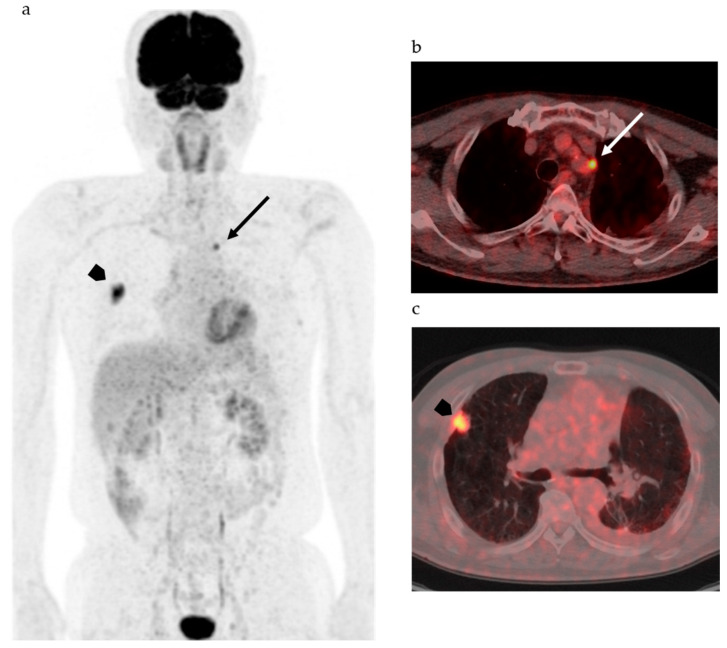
2-[^18^F] fluoro-2-deoxy-d-glucose positron emission tomography/computed tomography (FDG-PET/CT) of patients with non-small cell lung cancer. (a) Maximum intense projection image shows abnormal FDG uptake in the left upper mediastinum (arrow) and the right lung (arrowhead). (b) PET/CT shows increased FDG uptake in the lymph node adjacent to the left subclavian artery at the level of the upper mediastinum (arrow). (c) High FDG uptake is seen in the nodule at the right upper lobe (arrow head).
